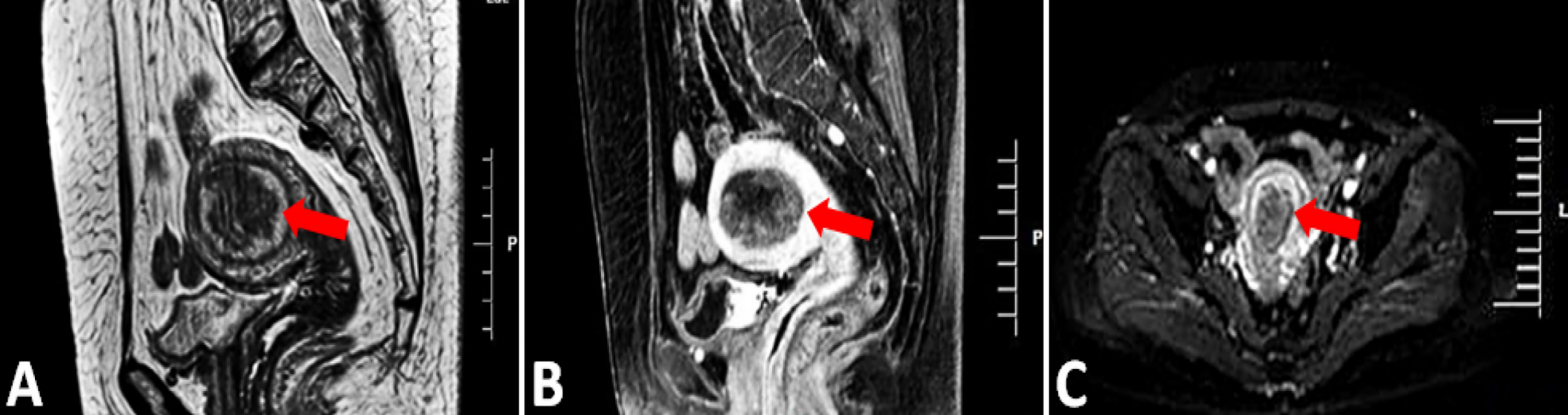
MRI, Magnetic resonance imaging. (A, B) Sagittal T2-weighted and T1 enhancement image showing the 5.2CM*3.5CM mass in the uterine cavity, the abnormal signal affected more than 1/2 of the myometrium (arrow). (C) Axial diffusion weighted image showing hyperintense endometrium (red arrow).
